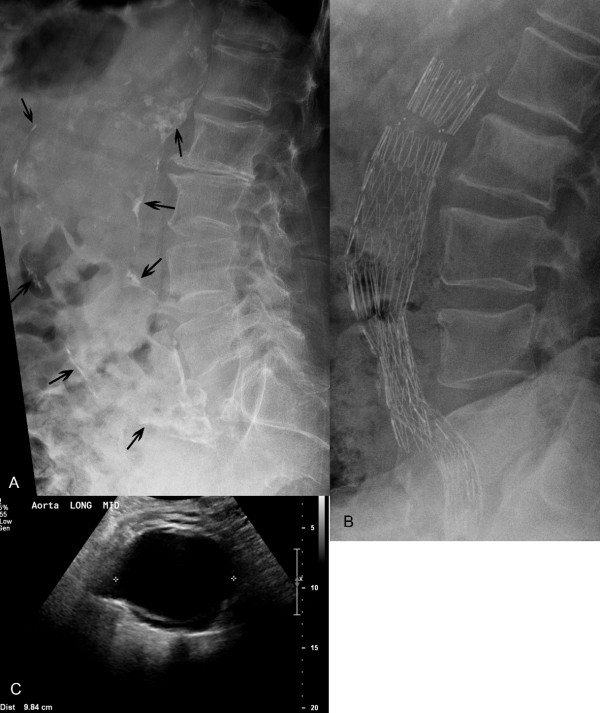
Abdominal Aortic Aneurysm. A. In this 68 year old man, a lateral radiograph reveals severe atherosclerotic plaques depicted as conduit wall calcification outlining a 9 cm diameter aneurysm (arrows) extending from the upper abdominal aorta to the iliac arteries near the lumbosacral junction. B. In another patient, observe the metallic mesh of an aortic and iliac artery graft. This patient also has skeletal metastasis with osteoblastic lesions within the L2 and L5 vertebral bodies. C. A longitudinal diagnostic ultrasound image through the center of an aneurysm (same patient as in A) documents that the diameter of the lumen at its maximum width is 9.84 cm. (Images courtesy of Lindsay J. Rowe, Newcastle, Australia).
